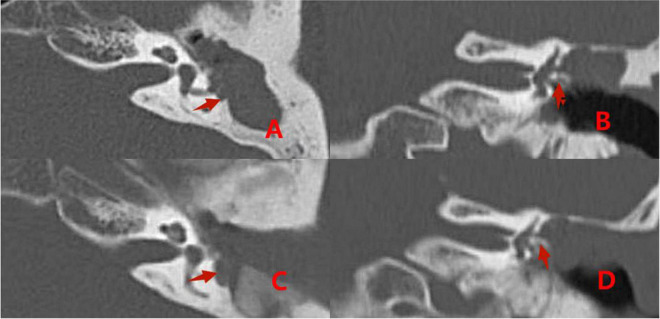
Pre- and postoperative CT images acquired from a patient with a type III labyrinthine fistula. Preoperative (A,B): The left cholesteatoma is adjacent to the bone destruction (the red arrows). Postoperative (C,D): The removal of the cholesteatoma, the abnormal shape of the external semicircular canal, and intraoperative fillers inside and adjacent to it (the red arrows).
